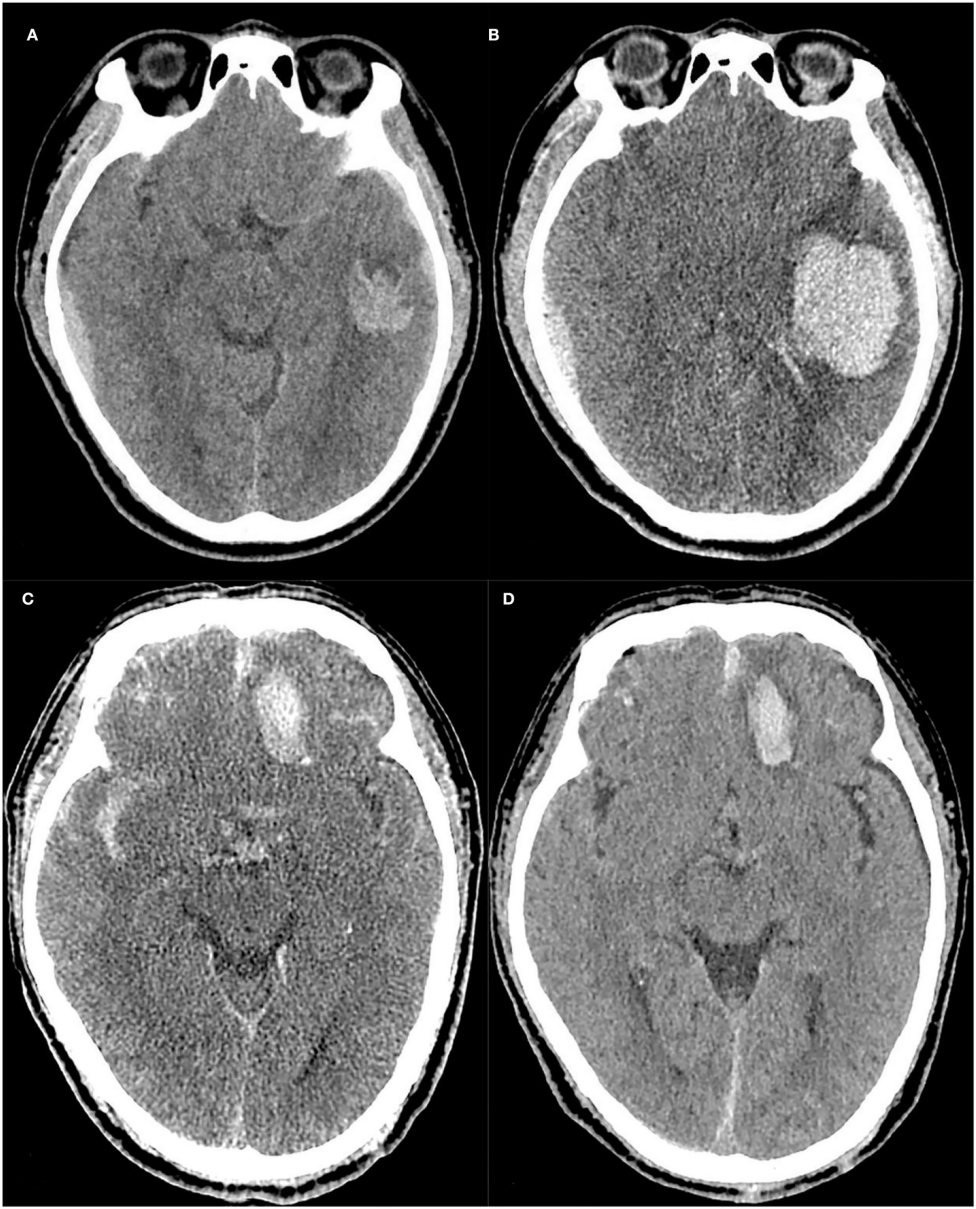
Case 1: A case with progressive hemorrhage, The baseline and follow-up CT images of a TBI patient (A,B), Scalp hematoma = 1, Fibrinogen = 2.68, Onset-to-CT time = 2 h, R-score = 1.925. The risk of PH calculated by the nomogram was approximately 94%, and the hematoma volume was expanded to approximately 42.9 ml on the follow-up 10-h CT compared to 10.5 ml on the baseline CT. Case 2: A case without progressive hemorrhage, The baseline and follow-up CT images of another TBI patient (C,D), Scalp hematoma = 1, Fibrinogen = 3.01, Onset-to-CT time = 6 h, R-score =-1.144. The risk of PH calculated by the nomogram was approximately 0.044%. At 17 h after trauma, the hematoma volume had not expanded.
